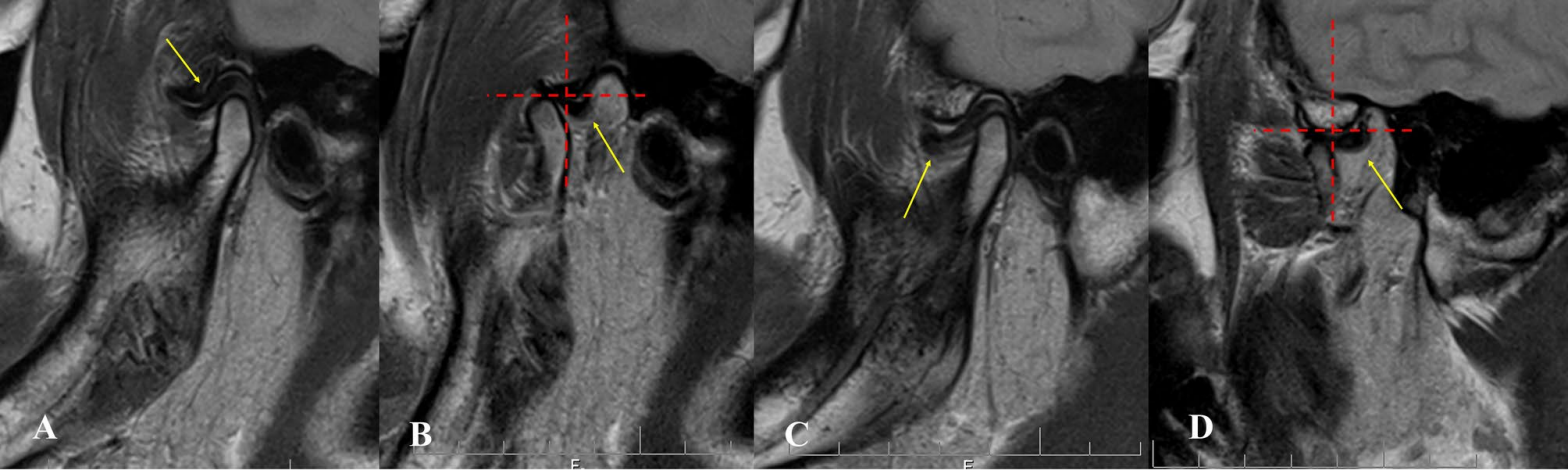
Oblique sagittal T1-weighted images represent Stage IV, showing a normal disk-condyle-fossa relationship (yellow arrow) in closed (A) mouth position with posterior disk displacement (yellow arrow) in open (B) mouth position. Oblique sagittal T1-weighted images represent Stage IV, showing anterior disk displacement (yellow arrow) in closed (C) mouth position with posterior disk displacement (yellow arrow) in open (D) mouth position.
